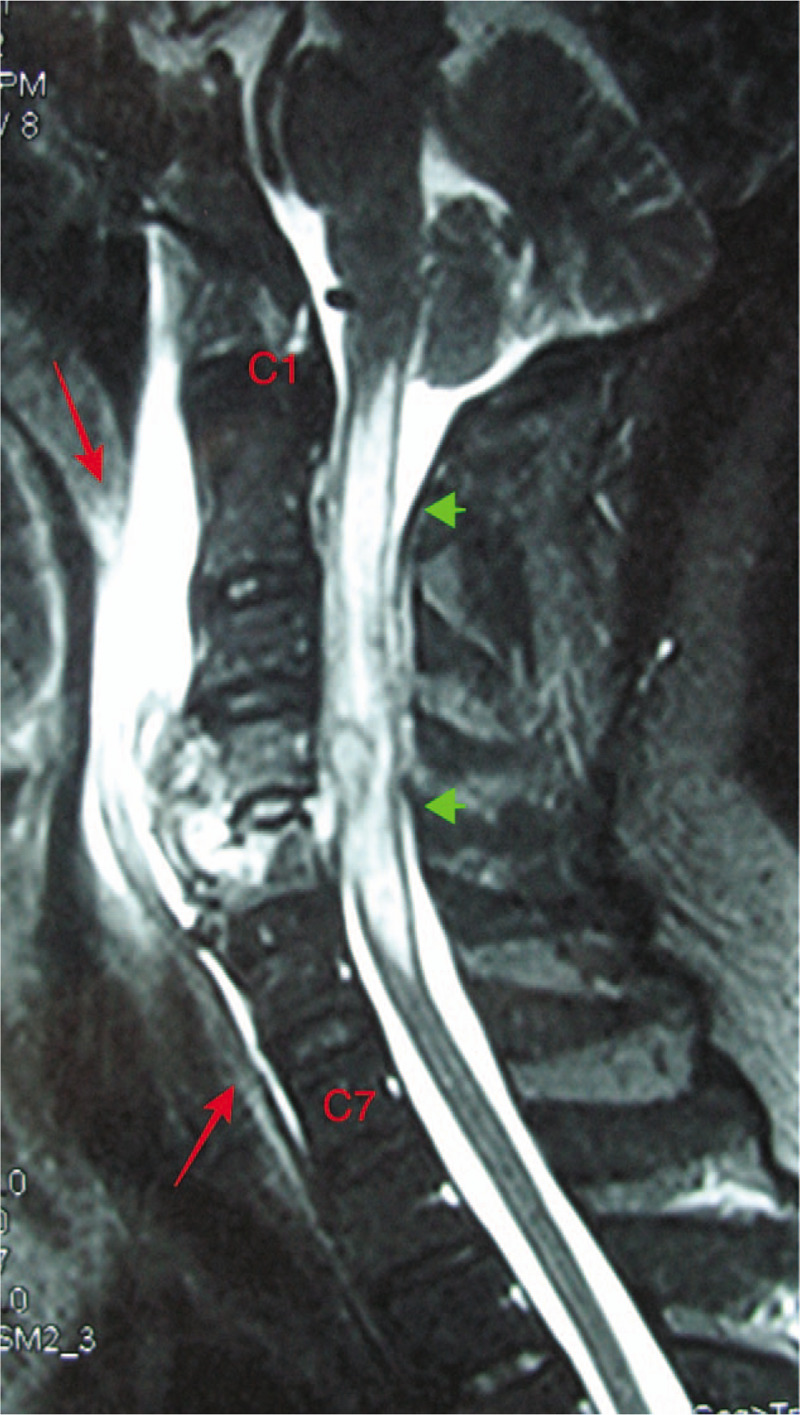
Sagittal cervical spine MRI on T2-weighted images showed that an increased diffusion signal in the C1–C6 spinal cord (arrowhead) and in the anterior soft tissue from the C1 to C7 cervical vertebral bodies (arrow). MRI = magnetic resonance imaging.
